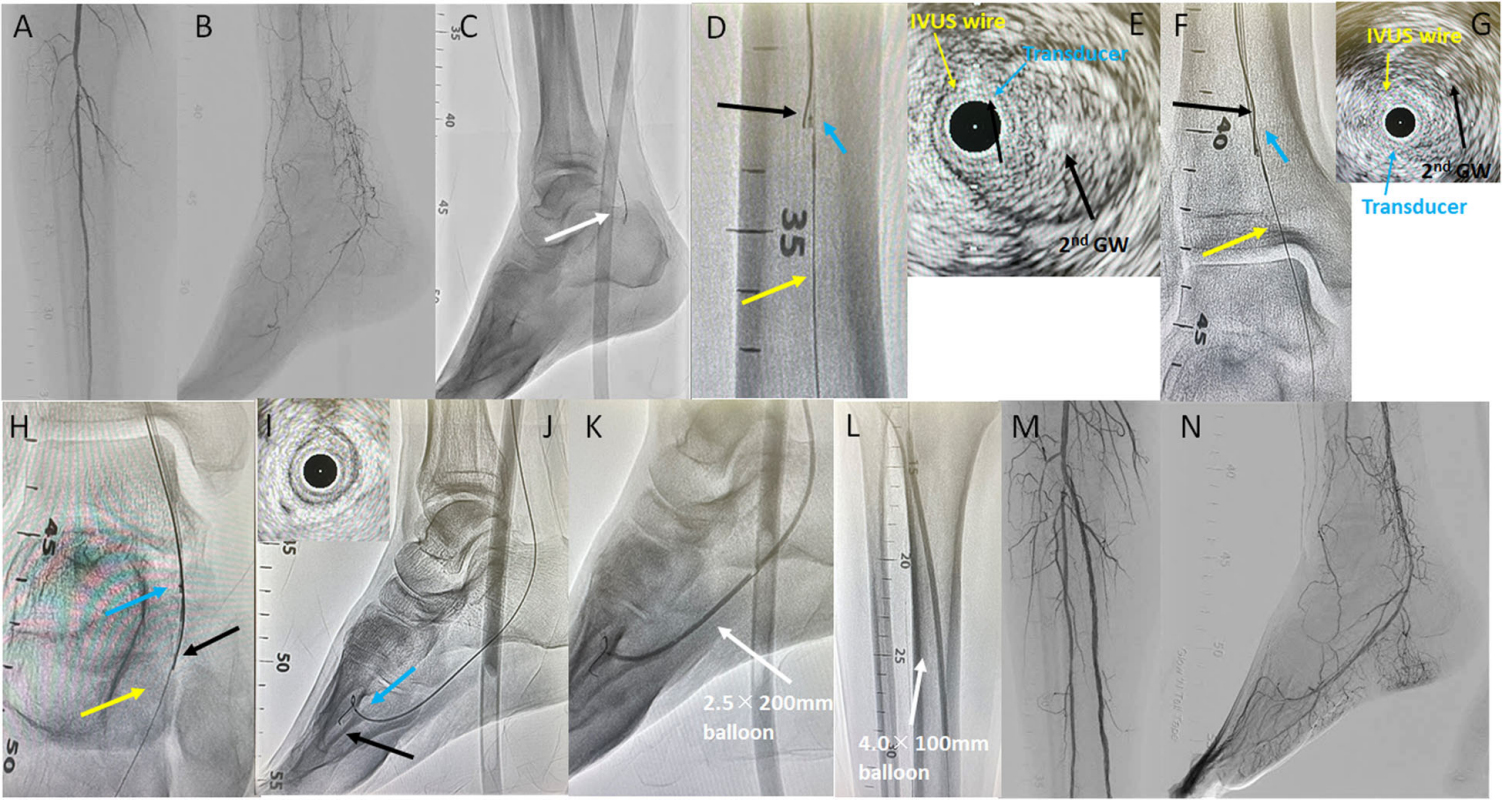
A, B Control angiography shows total occlusion of both tibial arteries, and very poor inframalleolar arteries. C A 0.014-inch guidewire (GW) is advanced into the subintimal space (white arrow: GW tip) in the posterior tibial artery (PTA). D, F, H Intravascular ultrasound (IVUS)-guided parallel wiring (D: proximal-to-middle PTA; F: distal PTA; H: lateral plantar artery). Yellow arrow: 1st GW and IVUS catheter; blue arrow: IVUS transducer; black arrow: 2nd GW advanced into the intraplaque of the PTA. E, G IVUS findings of the chronic total occlusion (CTO) lesion in the PTA (E: proximal-to-middle PTA; G: distal PTA). Yellow arrow: IVUS wire; blue arrow: IVUS transducer; black arrow: 2nd GW in the intraplaque route. I IVUS findings in the distal part of the lateral plantar artery. The IVUS transducer is almost in the center of the vessel. J The 2nd GW (black arrow) is advanced into the distal true lumen of the digital artery. Blue arrow: IVUS transducer. K A 2.5 × 200-mm balloon is dilated in the lateral plantar artery to the distal PTA. L A 4.0 × 100-mm balloon is dilated in the proximal PTA. M, N Final angiography shows sufficient dilation, antegrade blood flow, and small branches
